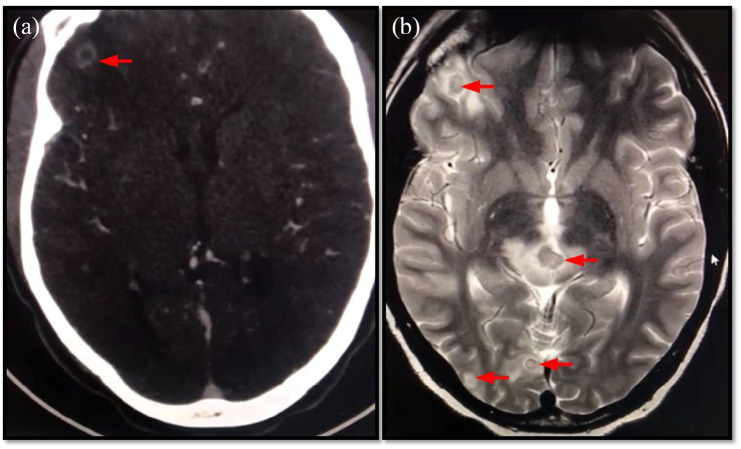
(a) CT brain showing ring-enhancing lesion on the left frontal lobe. (b)
